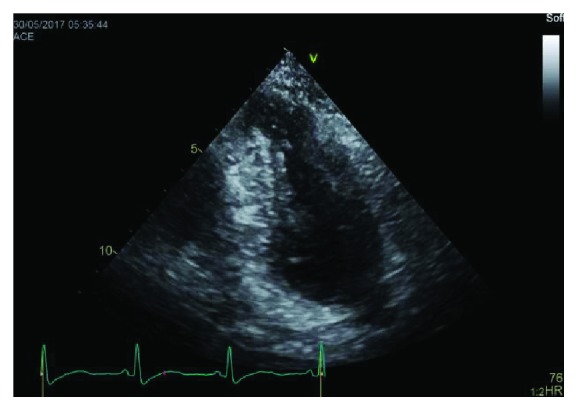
Apical 2 chamber view captured at end systole showing basal and apical akinesis with preserved contractility of the mid ventricular segments.
